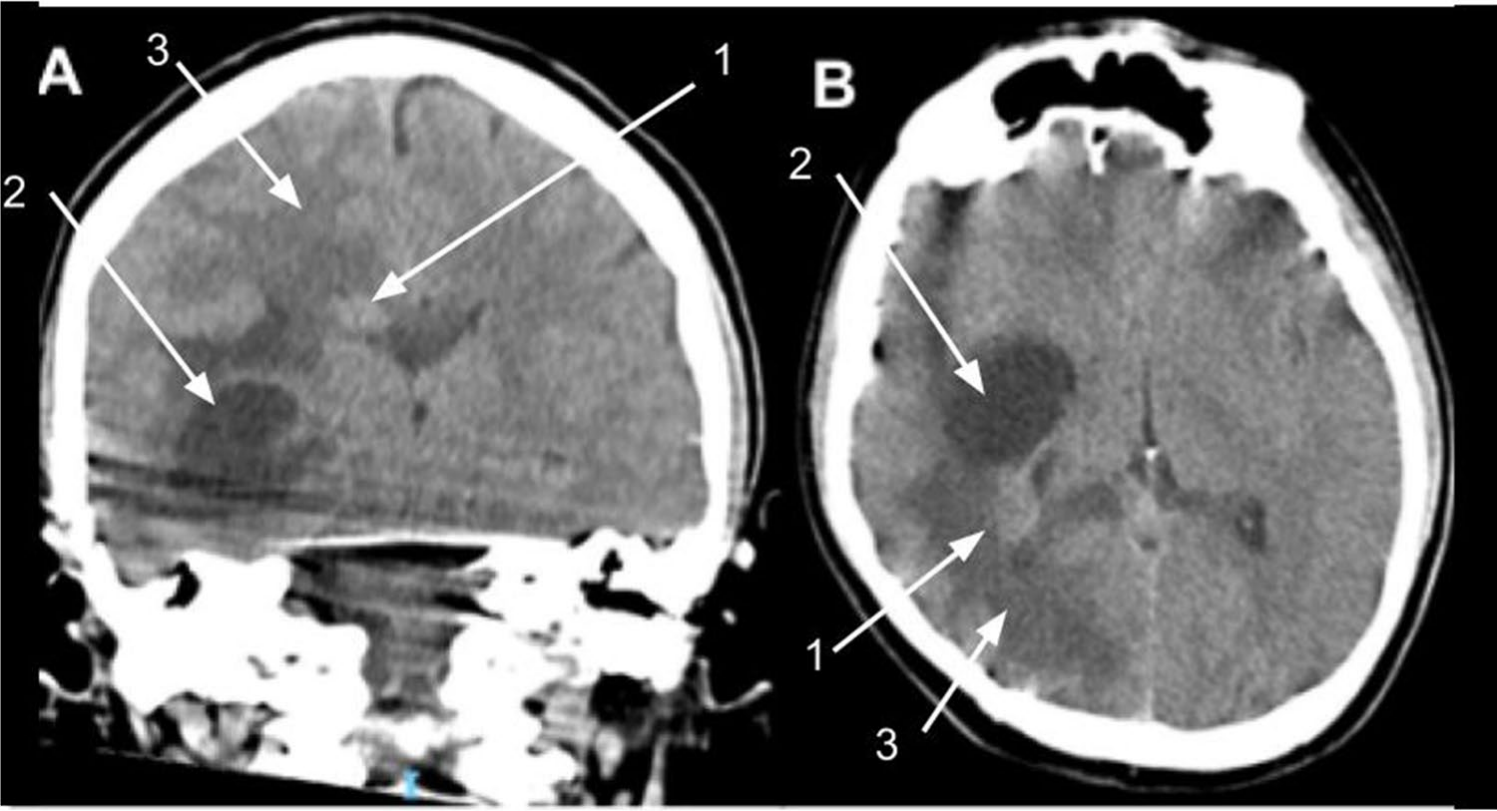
Computed tomography (CT) non-contrast brain images. A Coronal—mildly hyperdense infiltrating mass (1) in right lateral ventricle with apparent cystic temporal lobe component (2) and perilesional/ventricular edema (3). B axial—same lesion
